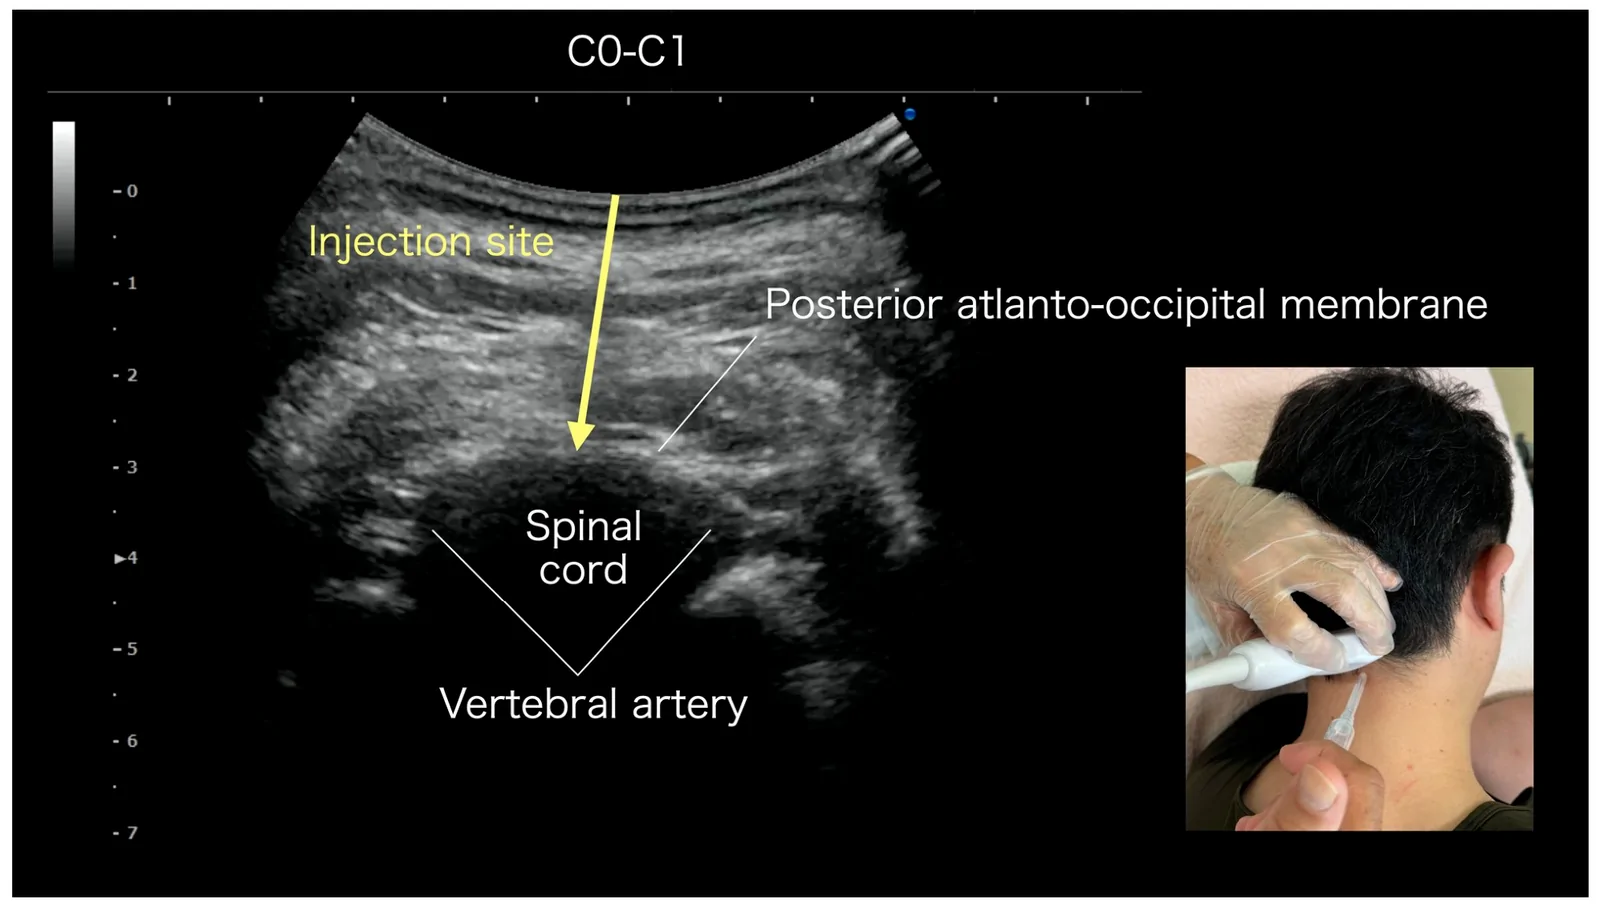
Upper posterior cervical release at C0–1 (POINT 11A, vertebral artery curvature; Advanced). Ultrasound image: Posterior atlanto-occipital membrane (PAOM), vertebral artery, and spinal cord are identified. The yellow arrow (injection site) indicates posterior advancement to the PAOM and release of the perivascular fascia around the vertebral artery curvature, together with densification along the PAOM. Selected in patients with dominant tenderness in the C0–1 region. The inset (bottom right) shows the patient position and probe placement.
